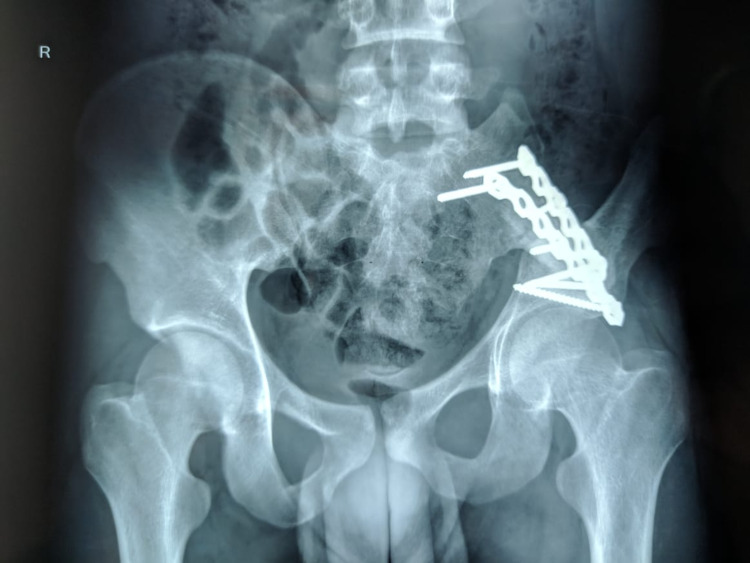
Two years postoperative radiograph showing union of graft.
